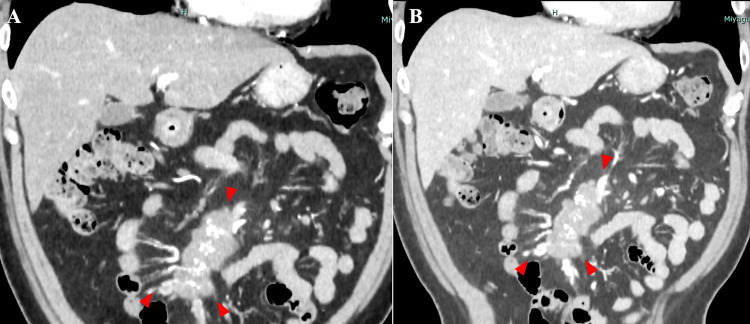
Coronal section of abdominal contrast-enhanced CT scan. The mass, indicated by red triangles, is depicted as hypovascular with poor contrast enhancement. No contrast effect was observed in either the arterial phase (A) or portal venous phase (B). CT: computed tomography
